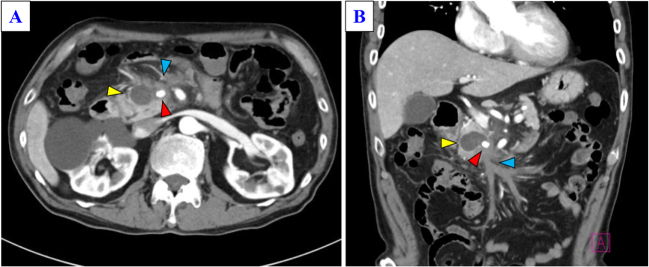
Contrast-enhanced computed tomography (CT) findings. (A) Axial and (B) coronal contrast-enhanced abdominal CT images at the initial presentation showing a 2.5 cm pseudocyst in the pancreatic head (yellow arrowhead) with a hyperintense area suggestive of intracystic hemorrhage (red arrowhead). The superior mesenteric vein (SMV) was extensively thrombosed (blue arrowhead).
